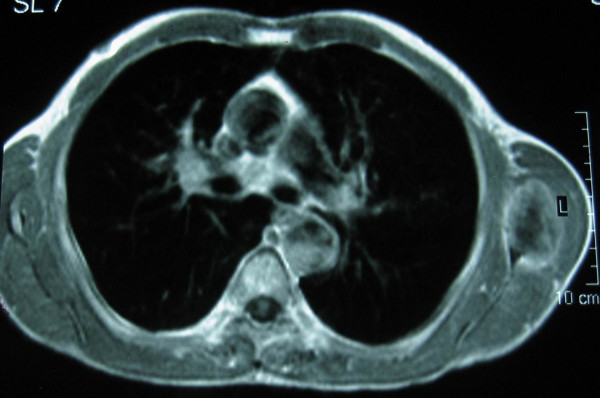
MRI image established the tumor measuring 45 × 45 mm.
